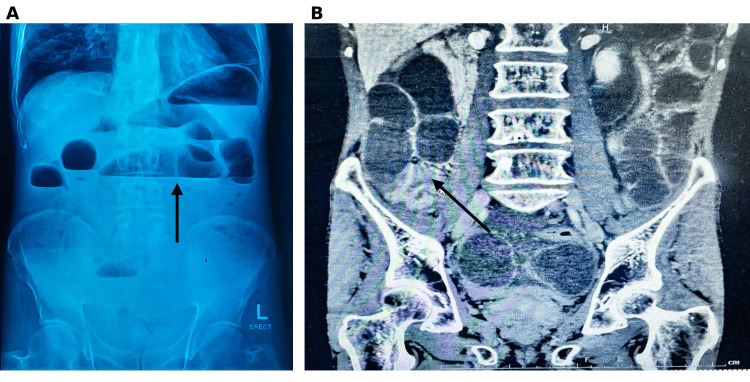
(A) Upright abdominal radiograph demonstrating multiple air-fluid levels; (B) contrast-enhanced computed tomography (CT) showing dilated bowel loops with a transition point suggestive of closed-loop obstruction.
